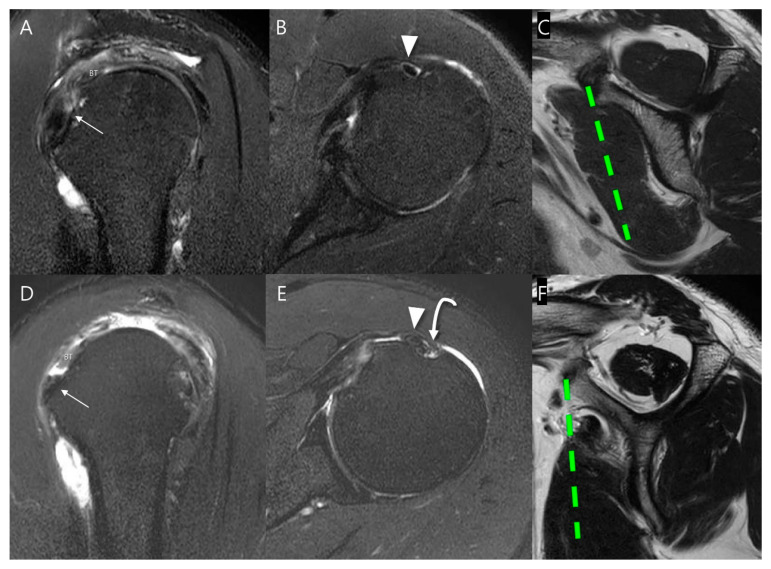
Representative MR images of Yoo type IIA (A–C) and IIB (D–F) subscapularis tendon (SSC) tears: (A) T2-weighted oblique sagittal fat-suppressed image in a patient with a Yoo type IIA lesion demonstrates a partial thickness tear of the SSC with ≤50% detachment from the first facet (arrow). (B) T2-weighted oblique axial fat-suppressed image shows an intact long head of the biceps tendon (LHBT, arrowhead) in the bicipital groove. (C) The T2-weighted oblique sagittal image shows no muscle atrophy. (D) T2-weighted oblique sagittal fat-suppressed image in a patient with a Yoo type IIB lesion demonstrates a partial thickness tear of the SSC > 50% detachment from the first facet (arrow). (E) T2-weighted oblique axial fat-suppressed image shows subluxation of LHBT (arrowhead) from the bicipital groove (curved arrow). (F) T2-weighted oblique sagittal image shows moderate muscle atrophy, especially in the upper half. BT, biceps tendon.
